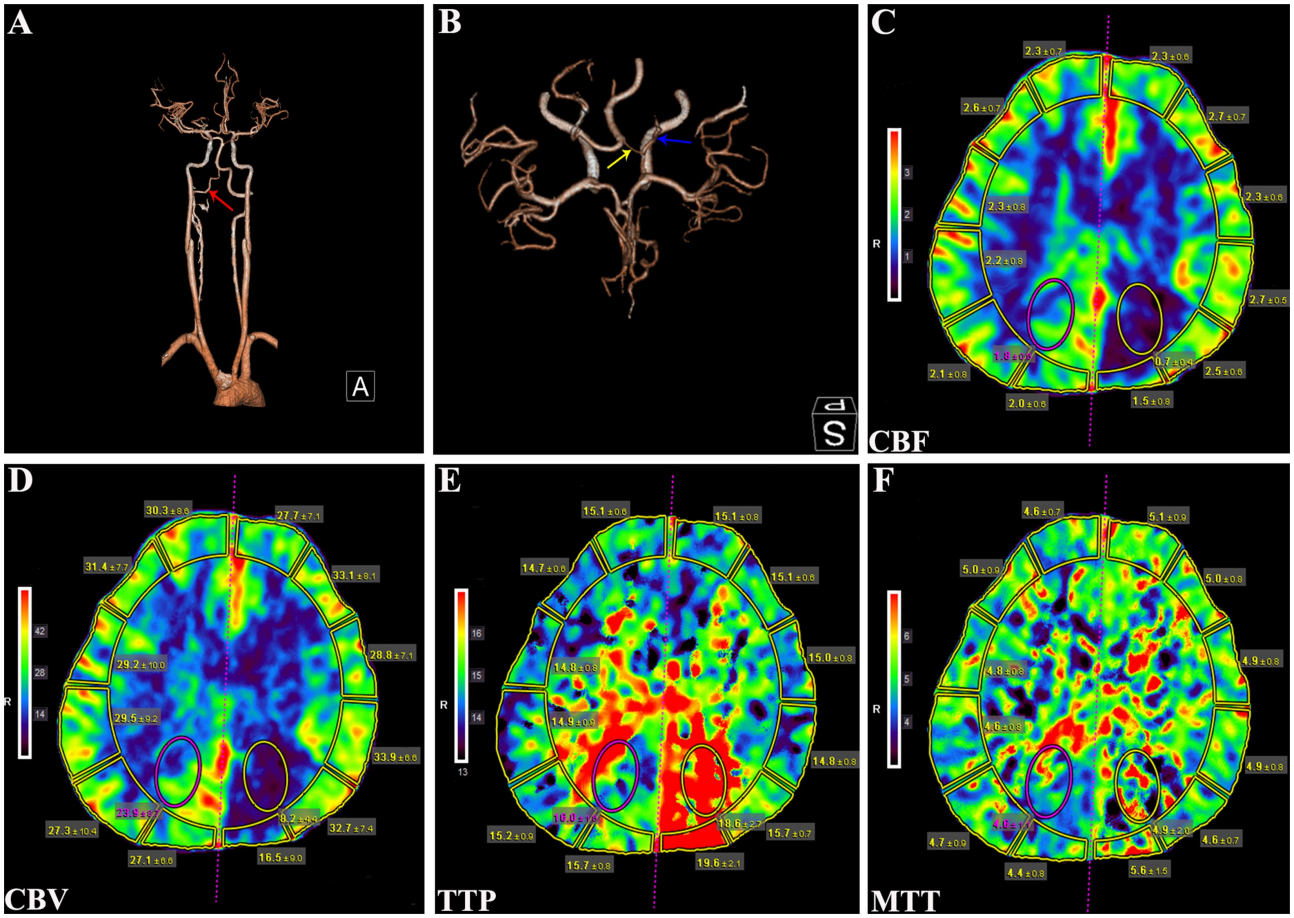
CTA and CTP analysis of the patient. (A) CTA showing a hypoplastic right VA (red arrow). (B) CTA revealing the left PCA originating from ipsilateral ICA with a hypoplastic P1 segment (yellow arrow) and a slenderly subsequent artery stem (blue arrow). (C) CBF, (D) CBV, (E) TTP, and (F) MTT parameters of CTP analysis demonstrating hypoperfusion in the left PCA supplying regions. CTA, computed tomography angiography; VA, vertebral artery; PCA, posterior cerebral artery; ICA, internal carotid artery; CBF, cerebral blood flow; CBV, cerebral blood volume; TTP, time to peak; MTT, mean transit time.
